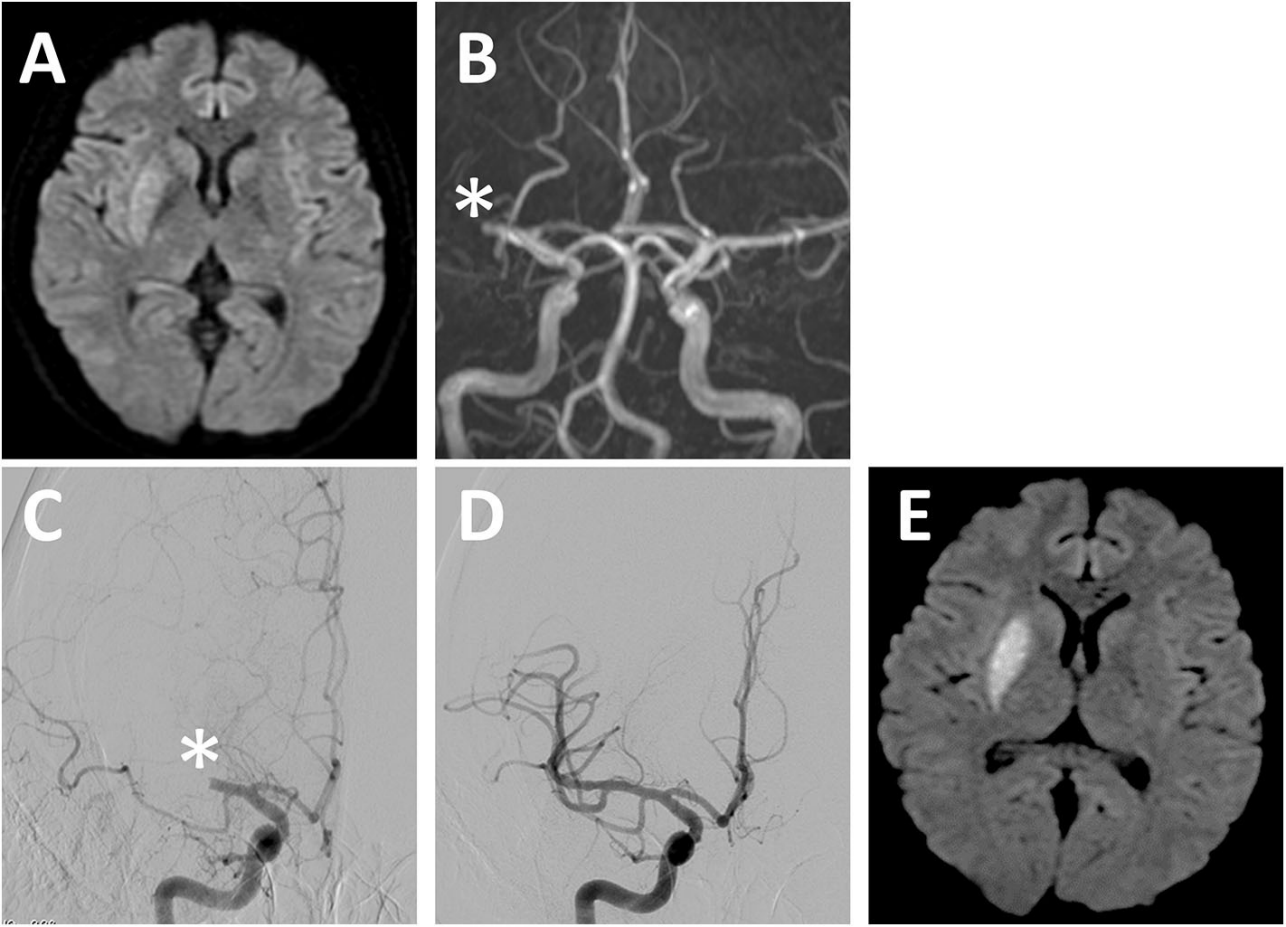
Representative case. (A) DWI and (B) MR angiography image on admission. MR angiography images at (C) pretreatment and (D) posttreatment with EVT. DWI on the following day of EVT (E). EVT, endovascular thrombectomy; DWI, diffusion-weighted imaging; MR, magnetic resonance. *Occluded vessel.
